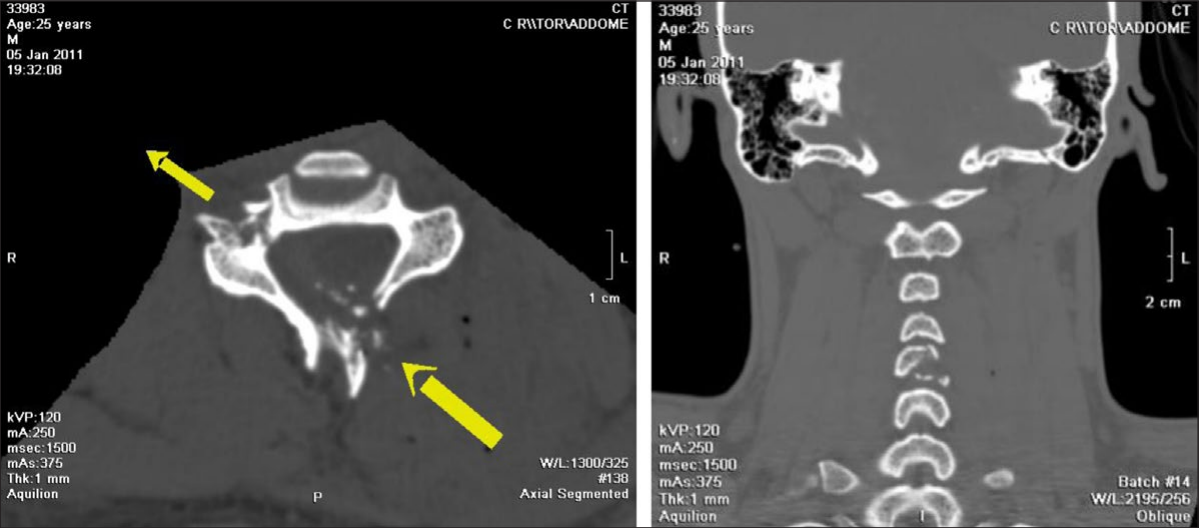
CT, axial (a) and coronal (b) sequences of the cervical spine. Fractures of the left posterior arch and of the proximal section of the C5 soma spinous process (input gunshot wound) and fracture of the right transverse C5 soma process (exit gunshot wound).
